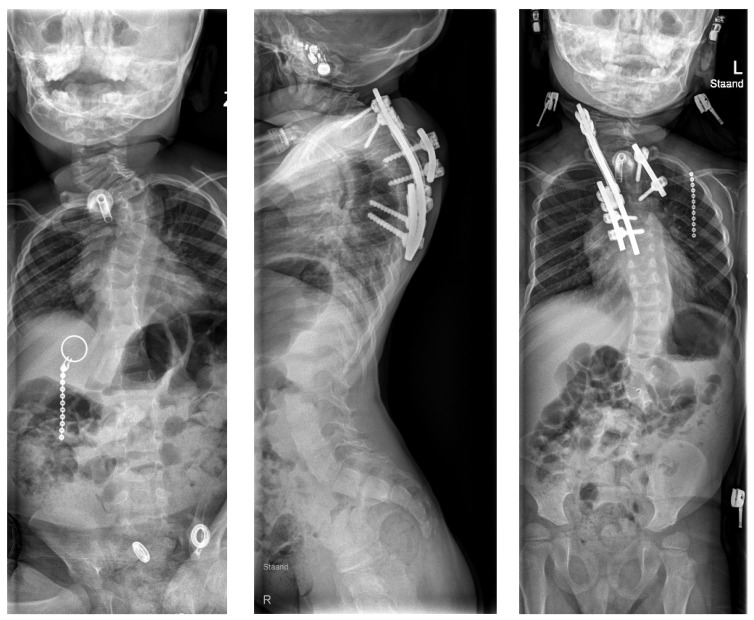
Case 2: preoperative AP sitting radiograph (left) and postoperative lateral (middle) and AP (right) standing radiographs.
